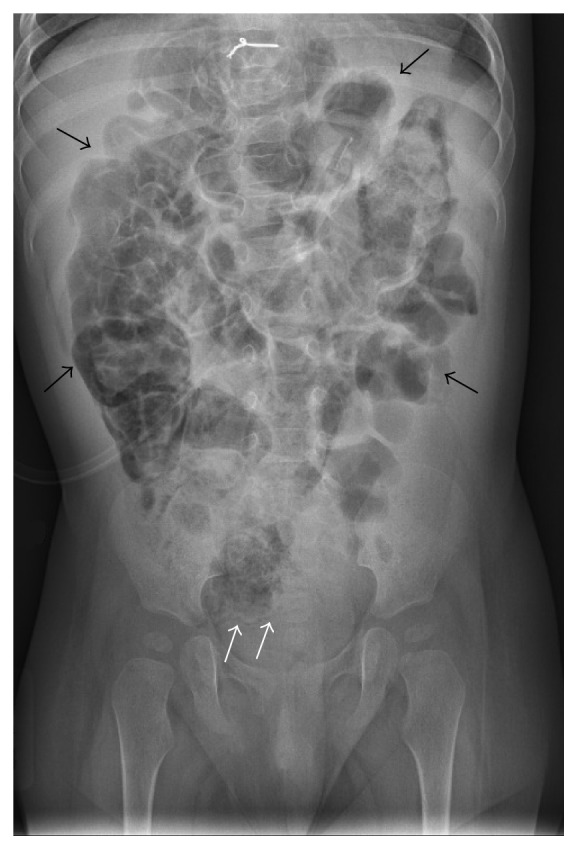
Abdominal X-ray (KUB) showing pneumatosis intestinalis, as evidenced by linear lucencies in the cecum (white arrows) and diffusely edematous bowel (black arrows).
